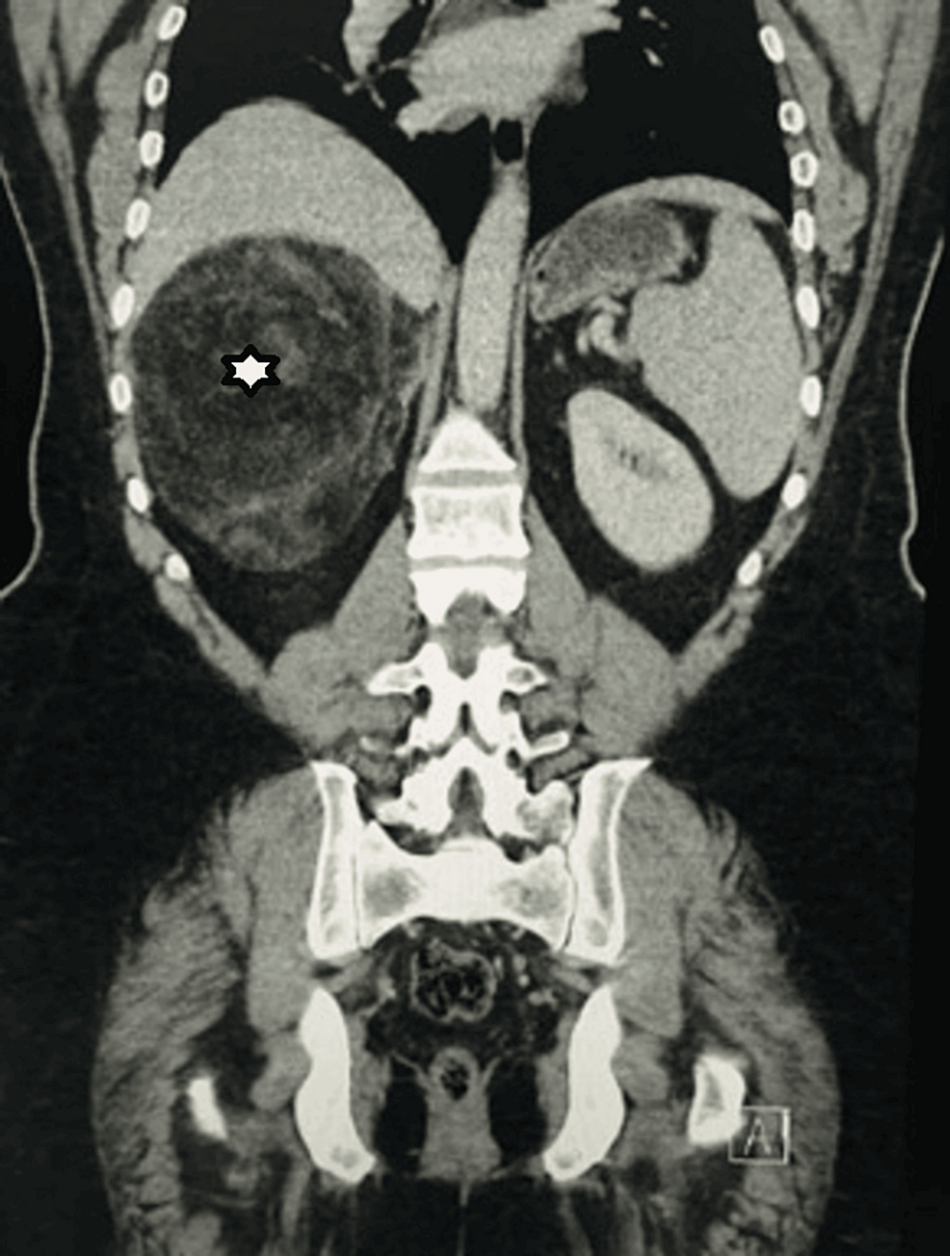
CT (Computed Tomography) image showing the largest mass in our study.
